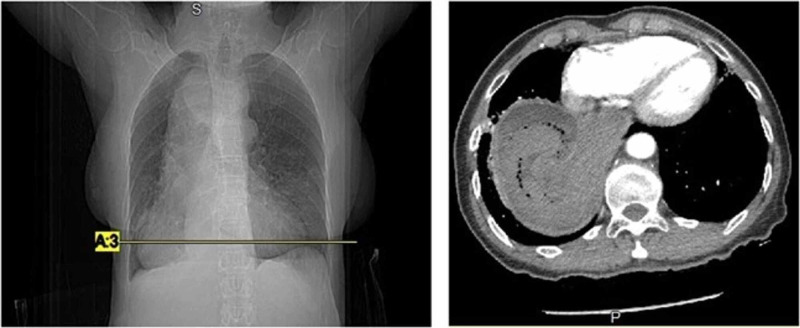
Computed tomography angiogram of the chest at a lower level with an axial view (left) and the corresponding coronal section (right) further showing a significantly dilated esophagus with small amounts of intraluminal fluid and gas
